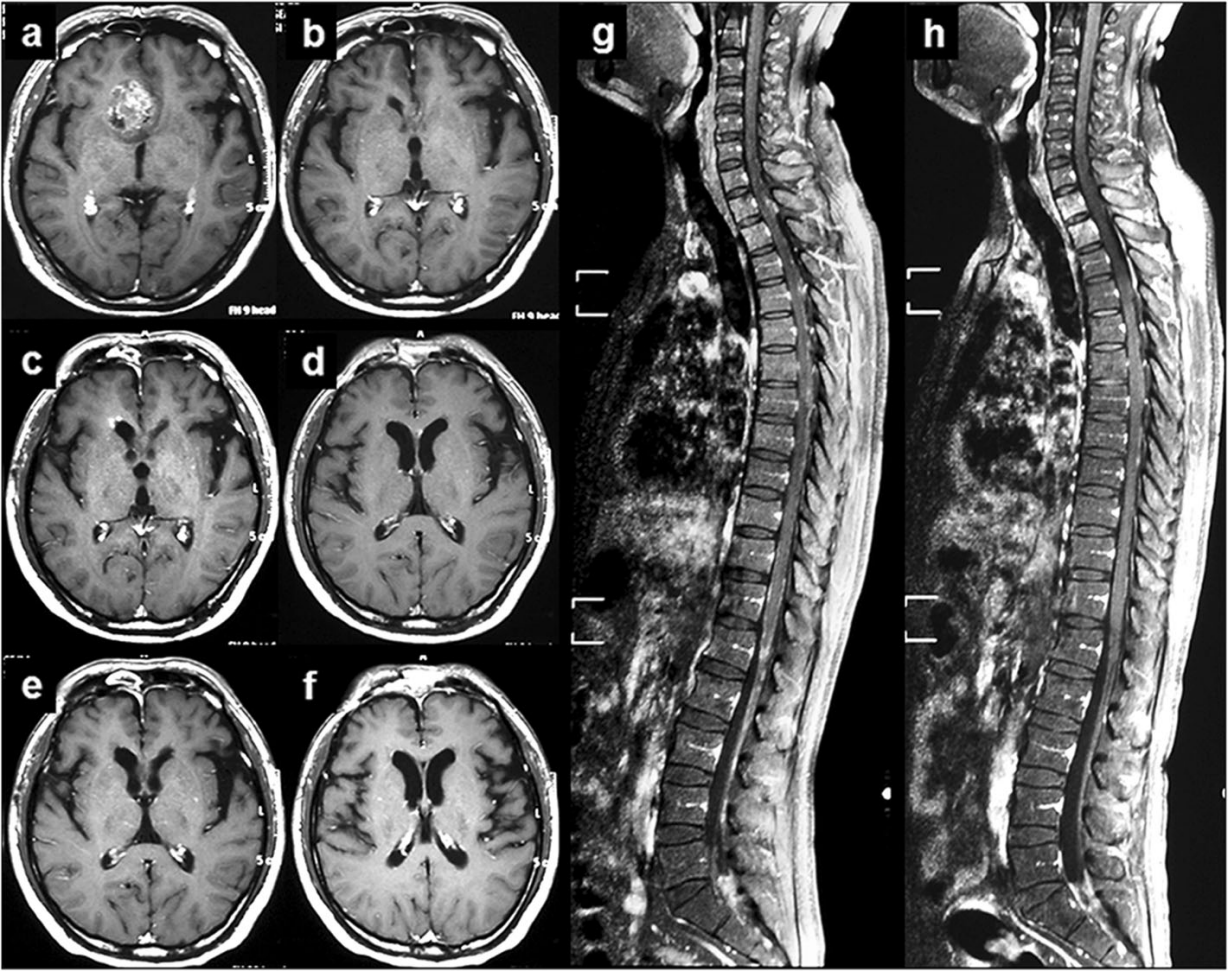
MRI of a typical case before and after treatment. a Evidence of a Gadolinium-enhanced lesion in the right frontal lobe before first surgery. b After surgery, chemoradiotherapy and adjuvant TMZ-based chemotherapy, the tumor got a complete response. c, d and g Eleven months after initial treatment completion, tumor recurrence was confirmed by MRI, which demonstrated widespread disseminated lesions in the frontal horn of right lateral ventricle, genu of corpus callosum and spine. e, f and h After 4 months of combined chemotherapy, the tumors were significantly decreased
